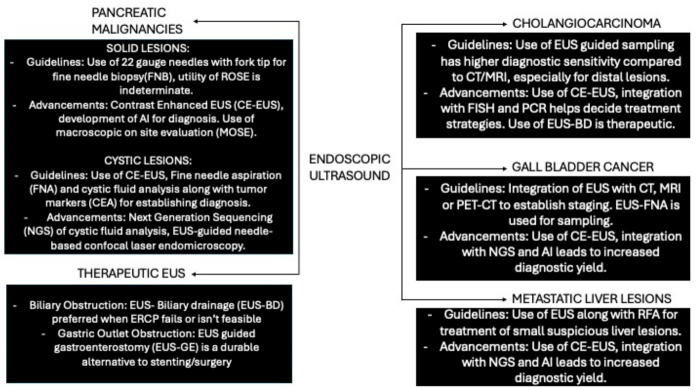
The image provides an overview of the evolving role of endoscopic ultrasound (EUS) in pancreatobiliary malignancies. EUS serves as a comprehensive platform for diagnosis, staging, tissue acquisition, and therapy. In pancreatic lesions, EUS-guided fine-needle biopsy (FNB), contrast-enhanced EUS (CE-EUS), artificial intelligence (AI), and advanced cystic fluid analysis improve diagnostic accuracy. In cholangiocarcinoma and gallbladder cancer, EUS enhances detection, nodal staging, and tissue acquisition, with CE-EUS, FISH/PCR, and next-generation sequencing (NGS) further refining diagnostic yield. EUS also plays a key role in detecting and sampling metastatic liver lesions, particularly small lesions missed by cross-sectional imaging. Beyond diagnosis, therapeutic EUS has emerged as a minimally invasive alternative for managing complications such as malignant biliary obstruction via EUS-guided biliary drainage (EUS-BD) and gastric outlet obstruction via EUS-guided gastroenterostomy (EUS-GE).
